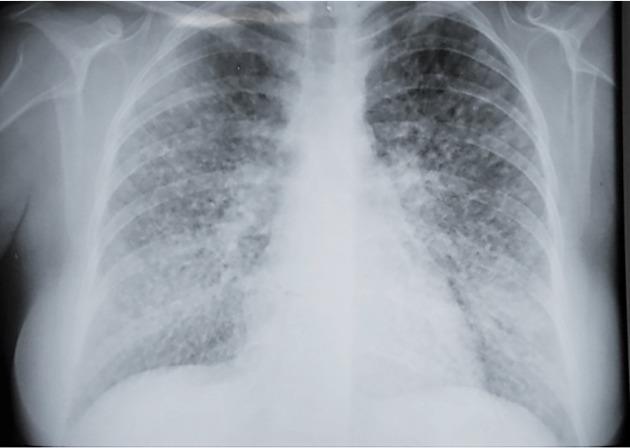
Chest X-ray showing a diffuse reticulonodular infiltrate and hilar
